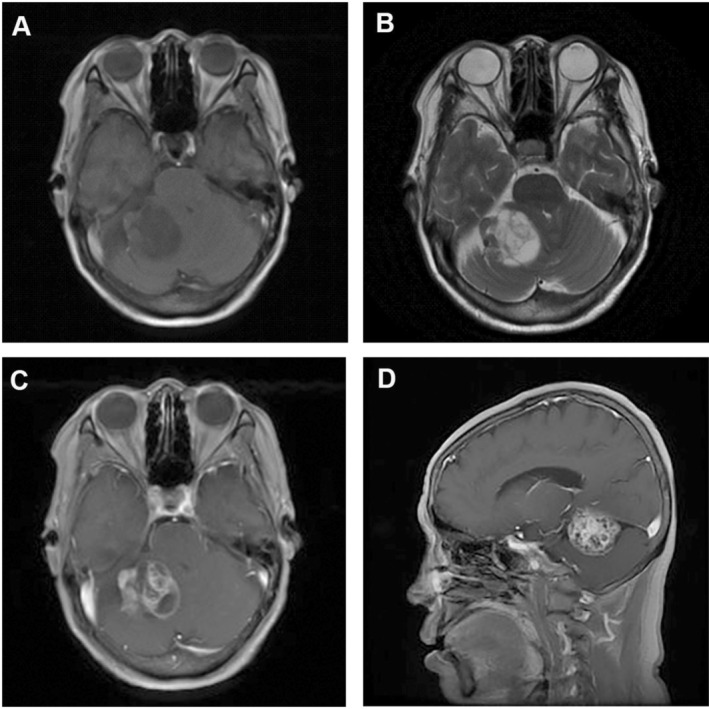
Cranial MRI showed a mass located in the tentorium cerebelli with heterogeneously isointense T1‐weighted (A) and T2‐weighted (B) image signals, and marked heterogeneous contrast enhancement on axial (C) and sagittal (D) T1‐weighted MRI with contrast enhancement.
